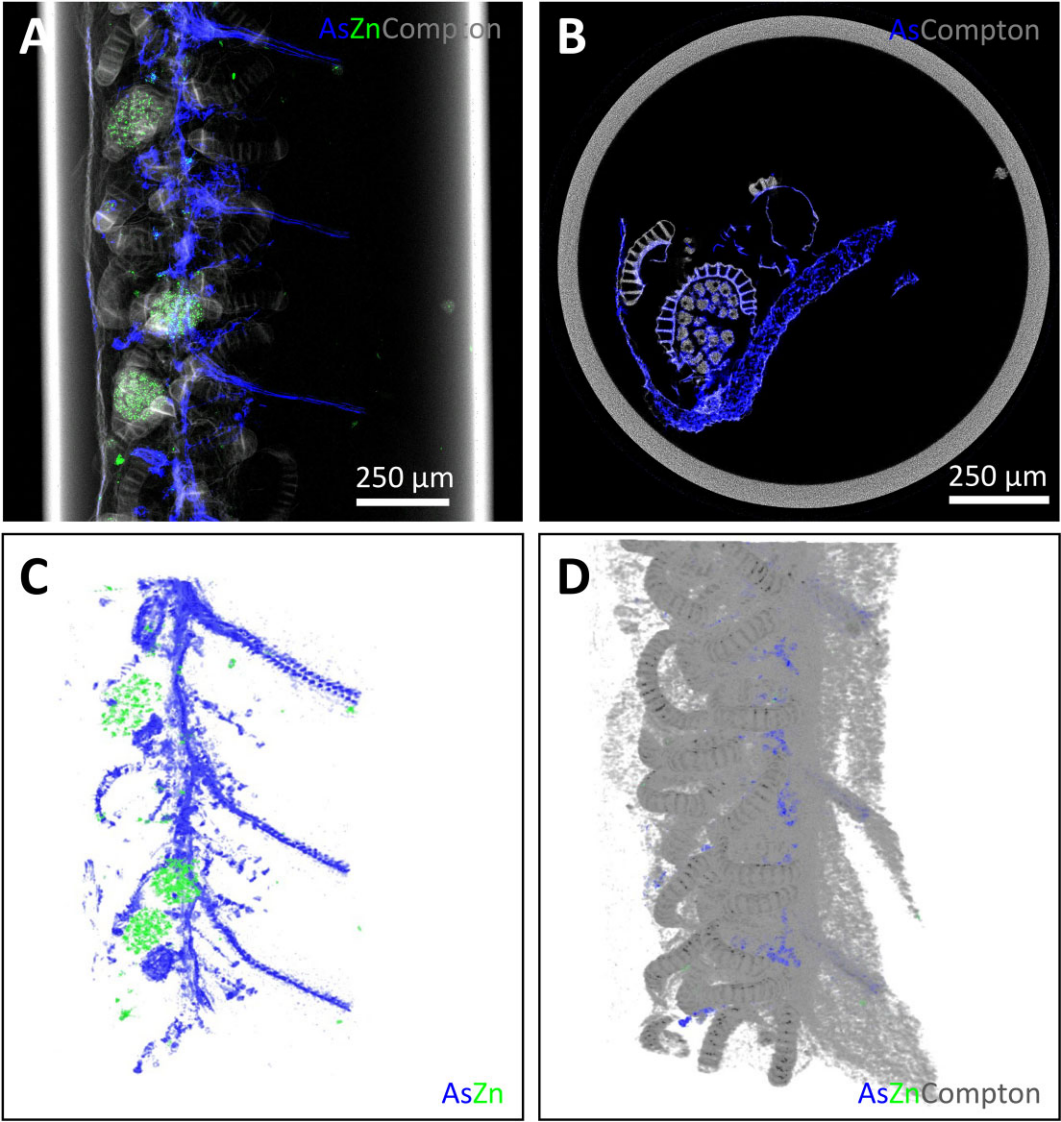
Synchrotron XFM scans of freeze-dried *Pteris vittata* pinna margin. A: 2D projection shows As (blue) predominantly in vascular bundles, and Zn (green) in sporangia (spore cases) clustered into sori, with these signals superimposed on the Compton image (gray). B: Single-slice XFM–CT reconstruction showing Compton (gray) and As (blue), revealing the lateral extent of the vascular bundles relative to the sori. C, D: Frames from within a rendered XFM–CT full 3D dataset of the same region in B, with the overall structure represented by the Compton signal, and As in vasculature and Zn in sori. (See Supplementary Information for video of full rendering). Reanalysed from source data presented in van der Ent *et al.*^25^
